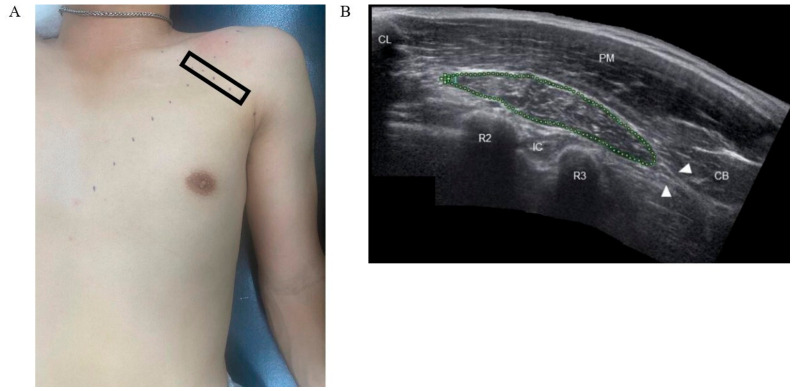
Placement of the probe and measurement on a panoramic image for the cross-sectional area of the Pm (A). Panoramic of pectoralis minor (Pm) cross-sectional area (B). PM, pectoralis major; CB, coracobrachialis; IC, intercostalis; Pm_CSA, pectoralis minor cross-sectional area; CL, clavicula; FAX, fascia axillaris (head arrows); R2, second rib; R3, third rib. The black box indicates the ultrasonographic probe position. The green dotted outline indicates the Pm_CSA.
